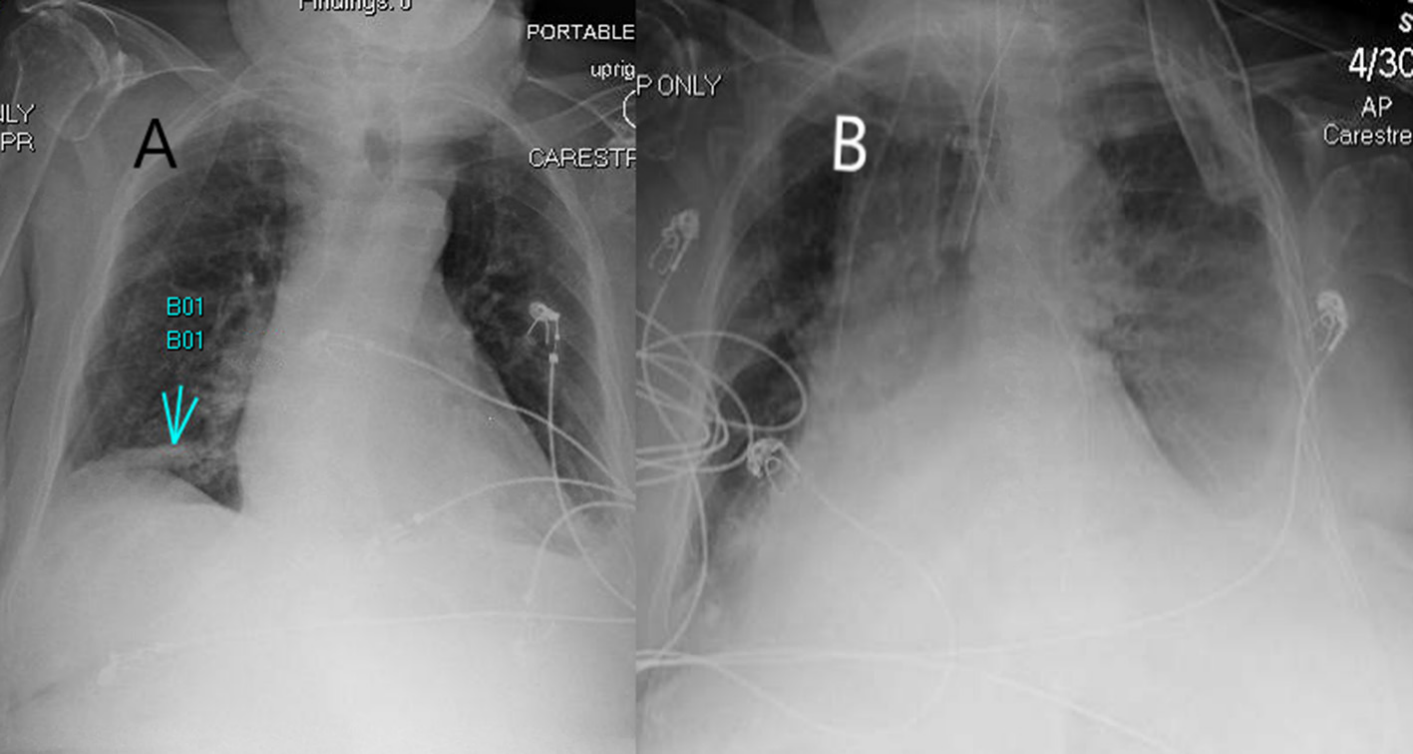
A 82-year-old woman presented to the emergency department with cough, shortness of breath, altered mental status. (A) X-ray showing a right lower lobe infiltrate (arrow) on day 1 and tested positive for COVID-19 using PCR. Later, due to worsening respiratory function and confusion, the patient was admitted to the intensive care unit and was intubated. (B) Image showing small left pleural effusion with worsening infiltrates.
